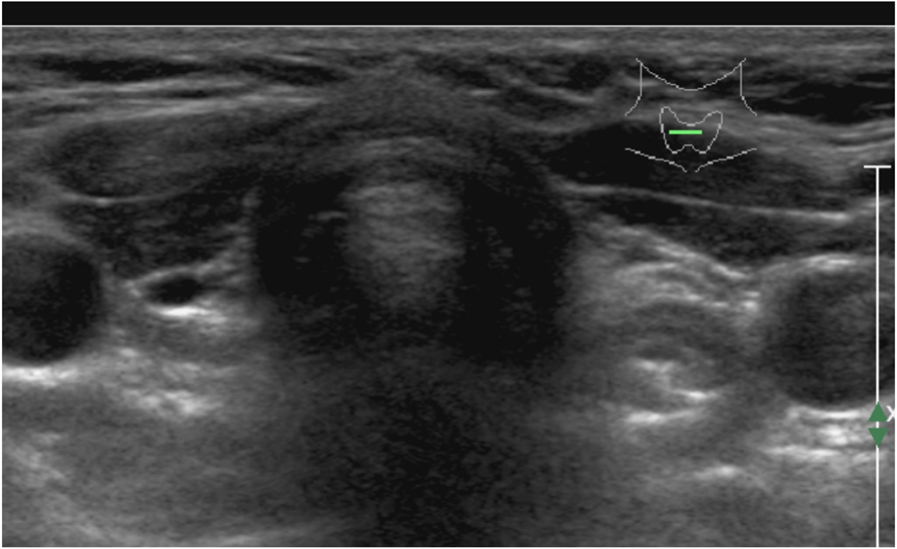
Ultrasonography of the neck showed absence of thyroid tissue
